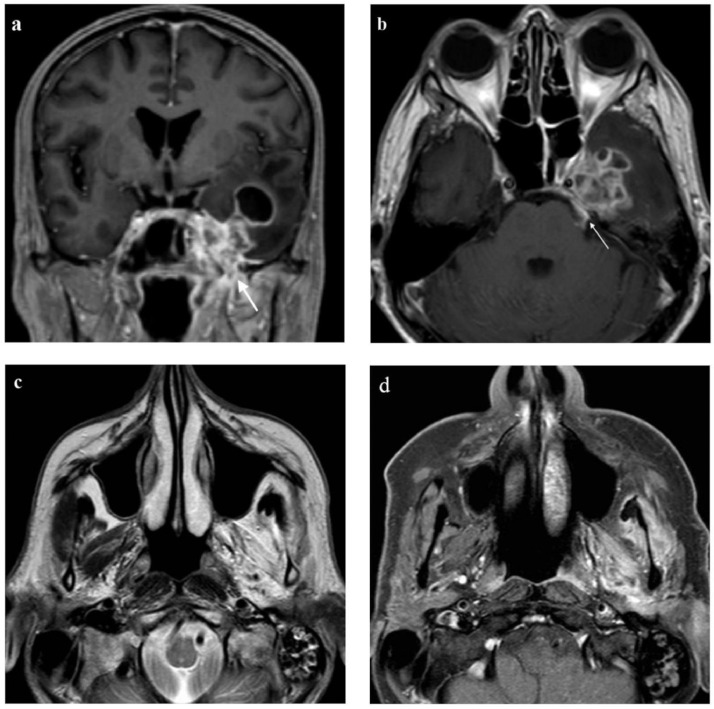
(Imaging on admission). (A) Contrast enhanced T1-weighted image in coronal plane reveals thickening and enhancement of the third branch of the trigeminal nerve in left foramen ovale (arrow). (B) Contrast enhanced T1-weighted image in axial plane shows thickening and enhancement of the cisternal part of the left trigeminal nerve (arrow). (C) Muscles of the left masticator space demonstrate a T2 hyperintense signal and (D) enhance after contrast agent due to denervation.
